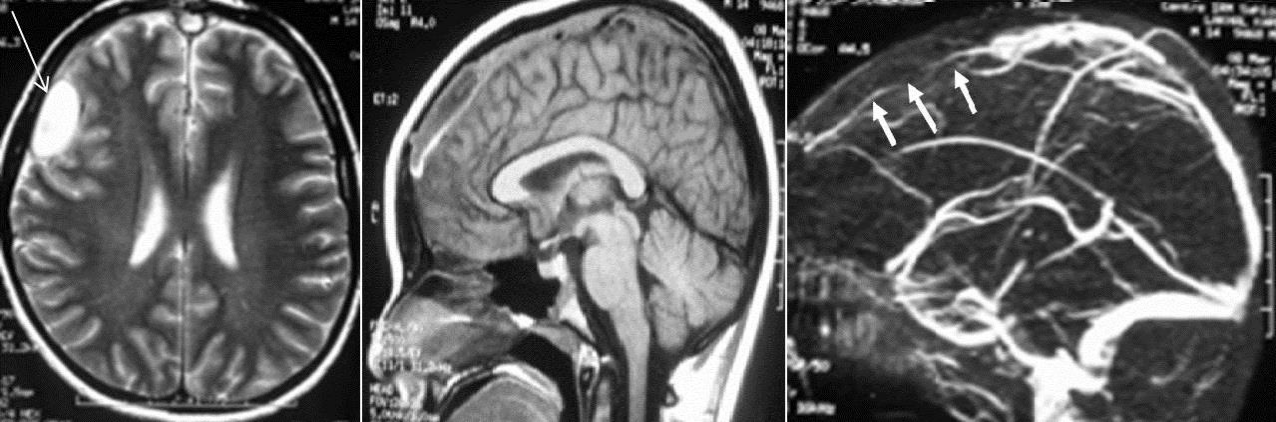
MRI and cerebral venous MRI showing a right frontal extradural empyema (long arrow) associated with a thrombophlebitis of the upper longitudinal sinus complicating frontal sinusitis (short arrows)
